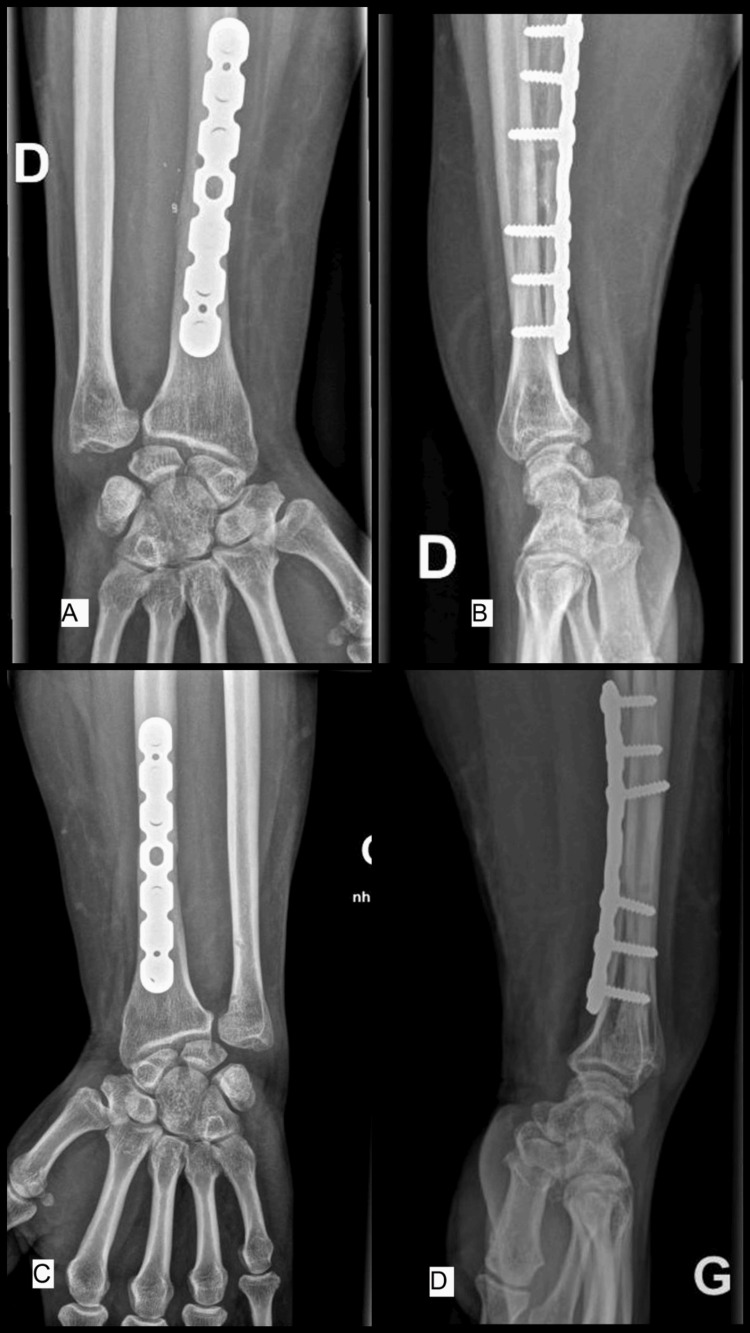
Postoperative X-rays Six-month postoperative AP (A) and lateral (B) views of the right wrist, and AP (C) and lateral (D) views of the left wrist, demonstrating consolidation of the radial fractures and stable DRUJ. DRUJ: distal radioulnar joint
